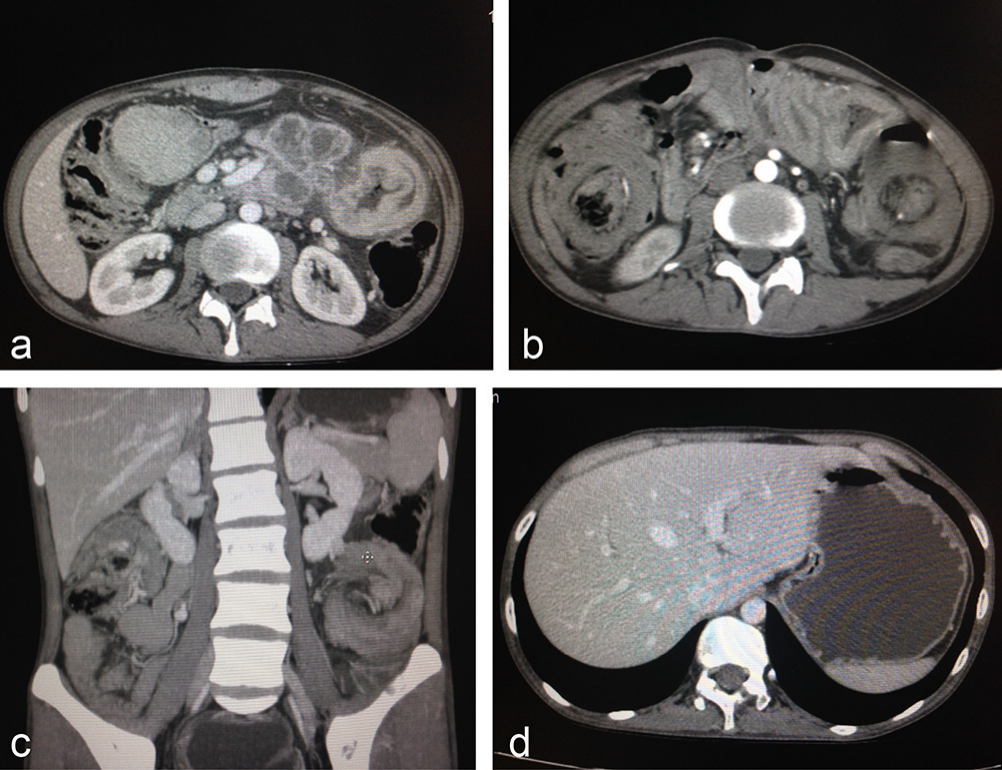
(a) Axial CT scan showing a heterogeneous peripherally enhancing mass in the mesentery just above the level of the renal hilum; the mass was not separate from the jejunal loops and was abutting the superior mesenteric artery, with maintained fat plane. (b) Reformatted oblique axial CT scan showing telescoping of the descending colon into the sigmoid colon, and the caecum into the ascending colon; however, the invaginated loop showed normal enhancement. (c) Coronal reformatted CT scan showing telescoping of the descending colon into the sigmoid colon, and the caecum into the ascending colon; however, the invaginated loop showed normal enhancement. (d) Axial CT image demonstrating multiple enhancing polyps in the stomach.
